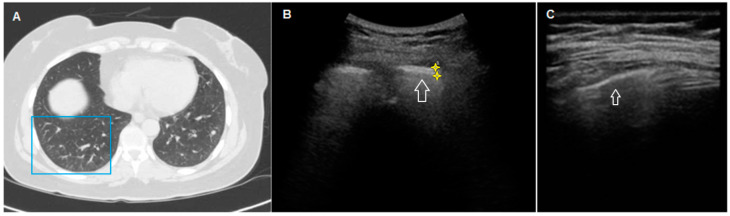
HRCT and TUS scan of a 54-year-old woman with familiarity for pulmonary fibrosis. In (A), HRCT scan shows a diffuse bilateral peripheral increase in pulmonary density (ground glass opacity) with widespread parenchymal nodular lesions (maximum size: 7 mm), in greater numbers in the lower lobes. In (B), TUS scan with a low-frequency convex probe (3.5–5 MHz) (in the chest area corresponding to the blue box in (A) shows a thickened pleural line (white arrow)). In (C), TUS scan with a high-frequency linear probe (8–12.5 MHz) at the same level does not show a clearly blurred and fragmented aspect.
